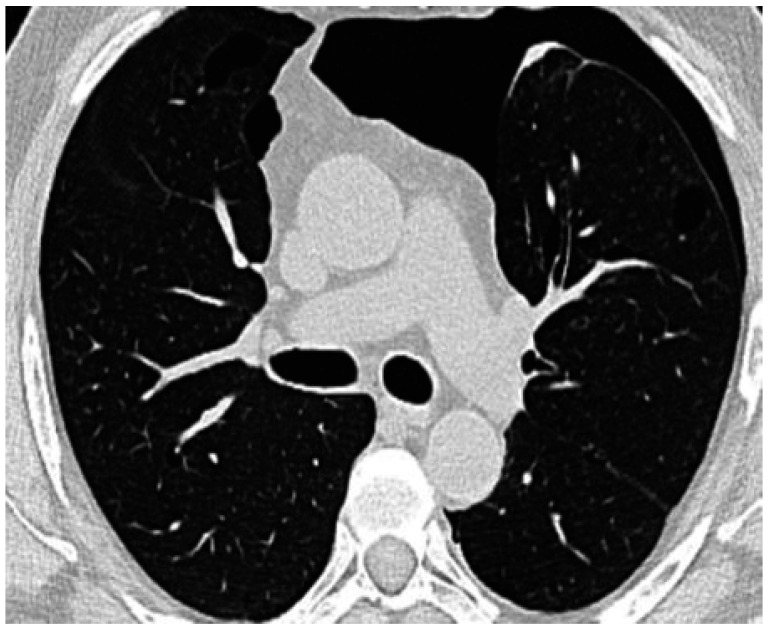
Chest CT, Case 6: bilateral cysts with greater involvement of the lower lung lobes.
